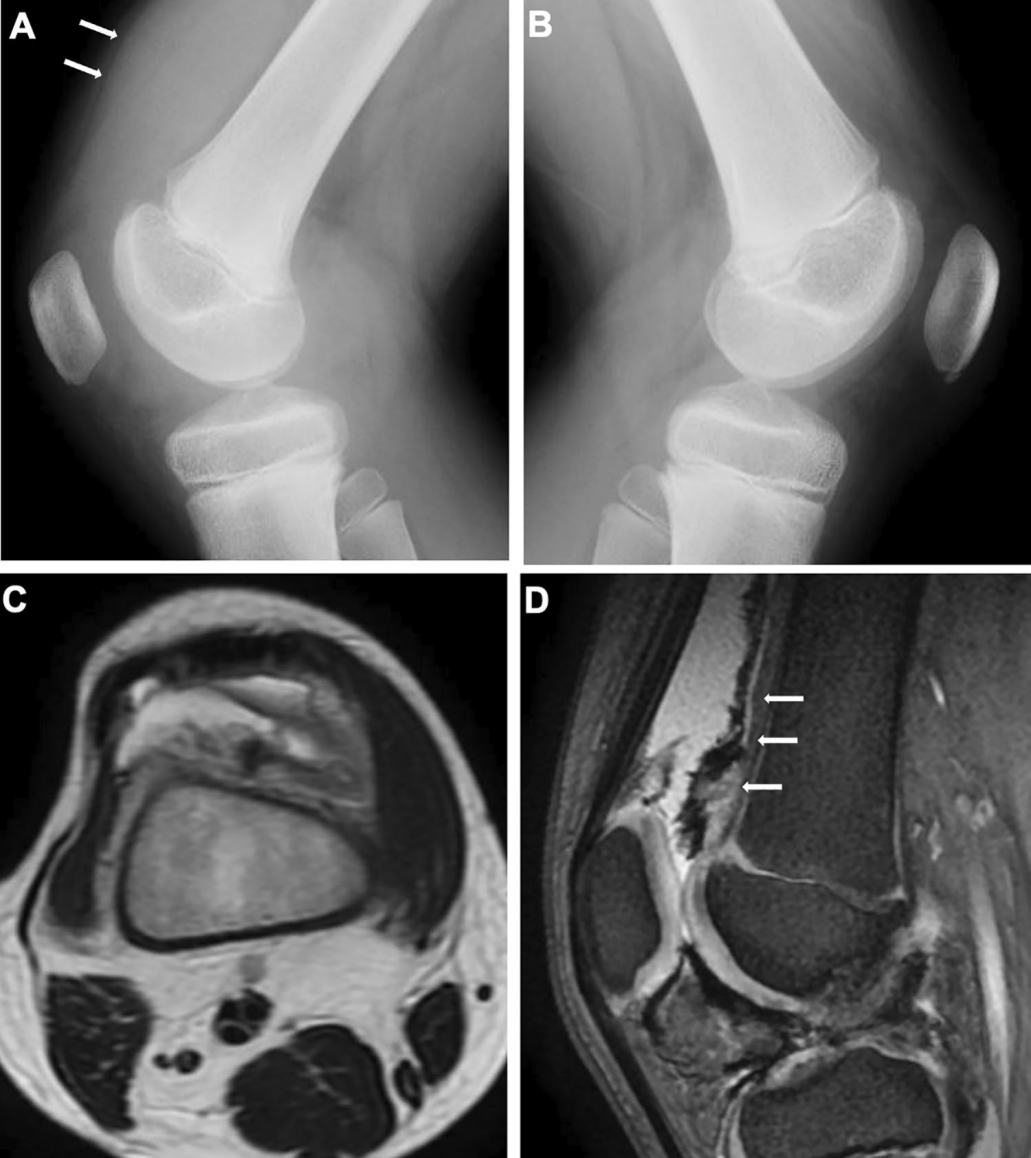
Tenosynovial giant cell tumor. A–D. A 9-year-old boy with swelling of the right knee. Lateral radiographs of the knees showed distention of the right suprapatellar bursa (arrows, A) and a normal left knee (B). Axial T2-weighted image (C) and sagittal gradient echo image (D) revealed synovial thickening and hemosiderin deposition lining the synovium. Hemosiderin is more clearly depicted on gradient echo image (arrows, D)
